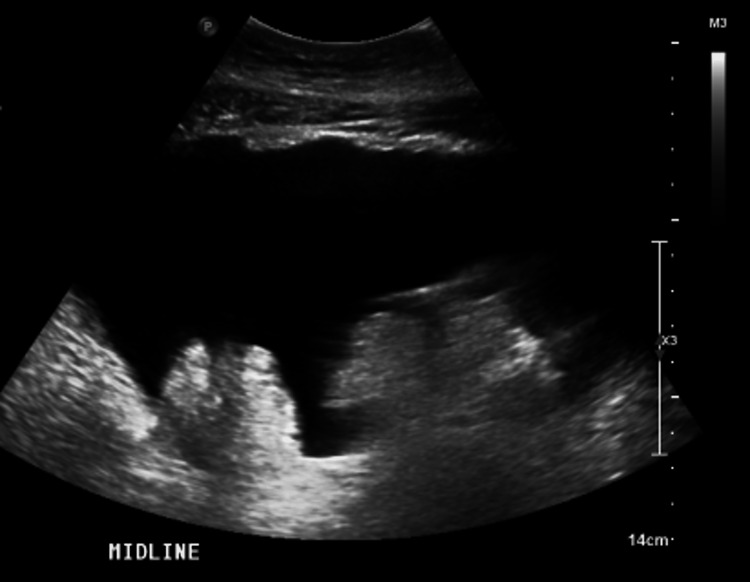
Significant ascites noted on abdominal ultrasound.
